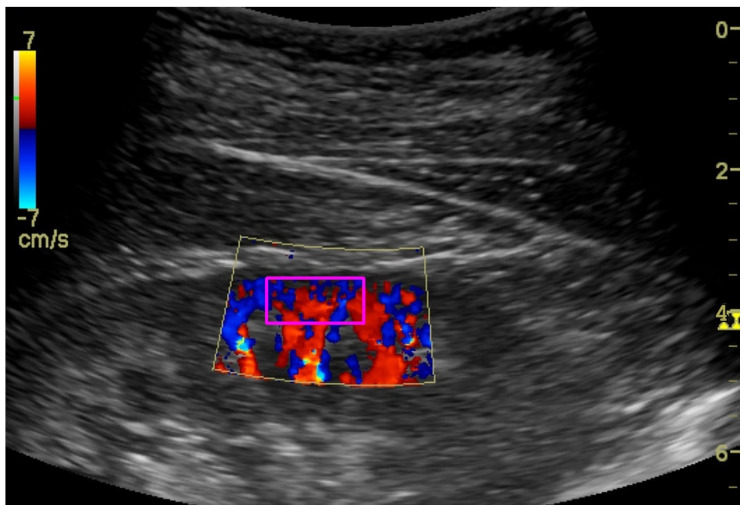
Ultrasound color Doppler visualization of renal cortex perfusion with marked (magenta rectangle) ROI.
